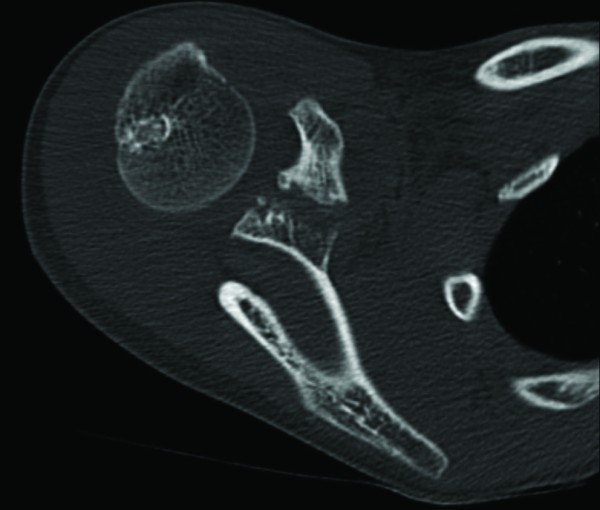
Axial CT image with a fracture of the base of coracoid process.
